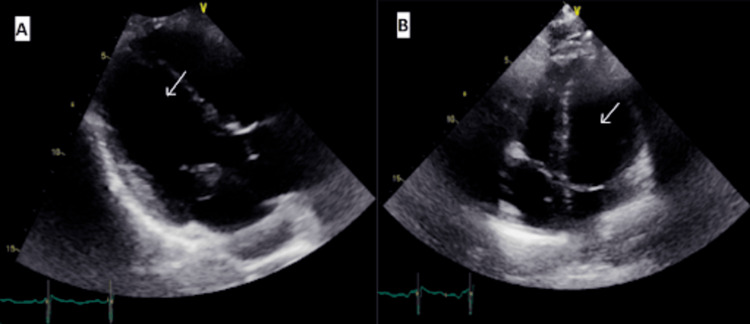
Initial transthoracic echo Parasternal long axis (A) and four-chamber (B) views show diffuse hypokinesis of the left ventricle (white arrow) with an ejection fraction of less than 30%.
